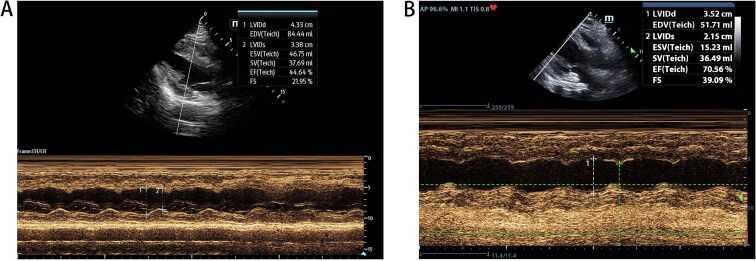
(A) Echocardiography of 10th May, EF = 44.64%. (B) Echocardiography of 18th May, EF = 70.56%.
